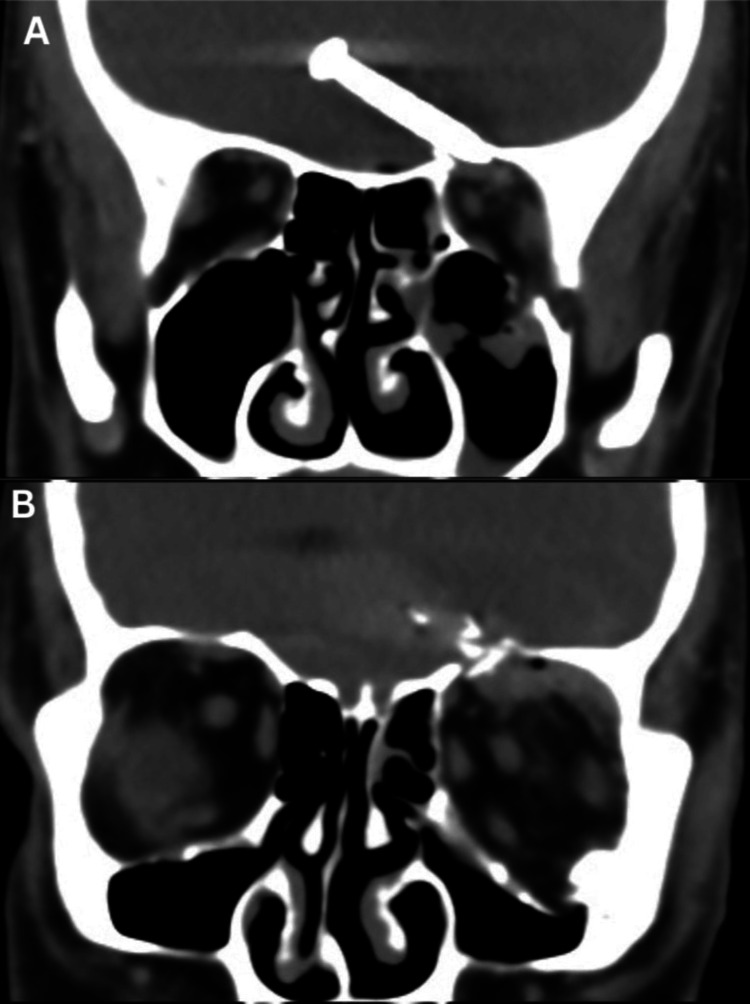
Non-contrasted CT brain (coronal view) showing the nail (A) and fractures of the left orbital roof and floor (B).
